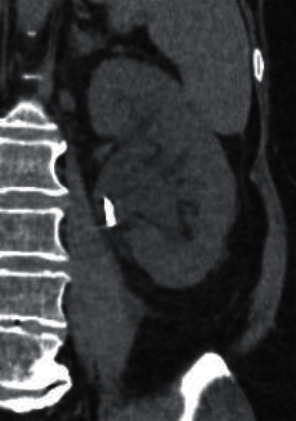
CT scan; persistent hydronephrosis despite DJ stent.
